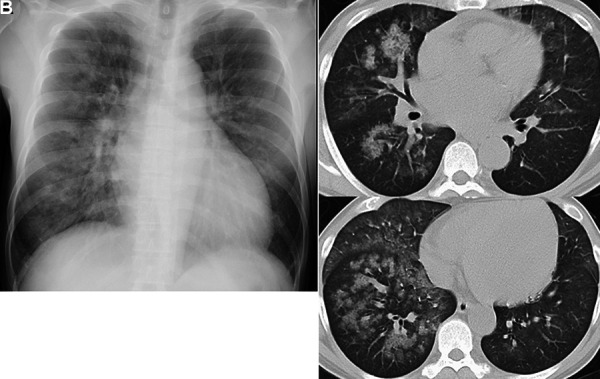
A: Chest radiograph showing extensive bilateral alveolar shadowing and cardiomegaly; cardiothoracic ratio 60%. B: High-resolution chest computed tomography on the first day revealed diffuse perihilar ground-glass attenuation with some areas of consolidation along the bronchial vascular bundle.
